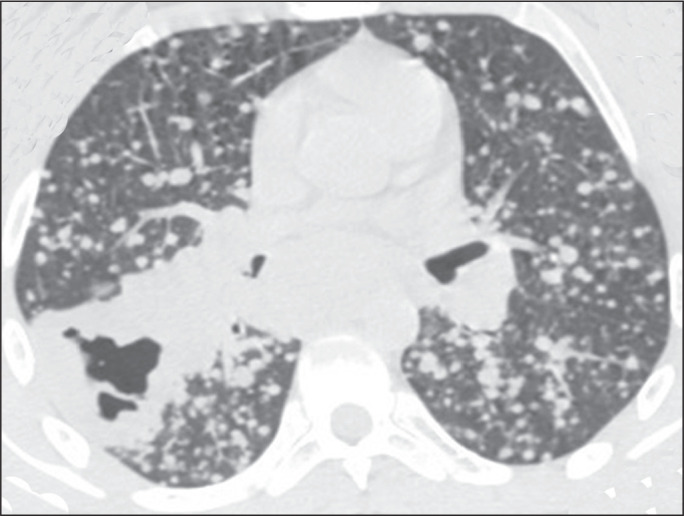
CT of a 29-year-old man, showing a cavitary mass, with internal
